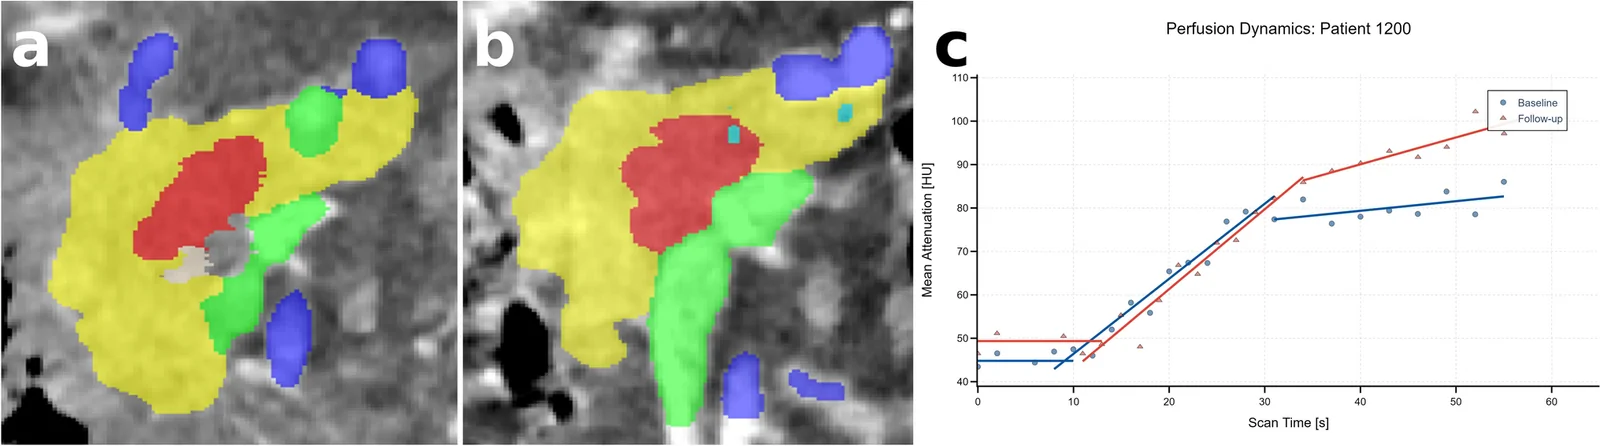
Representative CT perfusion images and analysis. A Baseline CTP image with AI-generated tumor segmentation (red),pancreas parenchyma segmentation (yellow), arteries (green) and vessels (blue). B Follow-up CTP at 3 months with corresponding segmentations. C Time-intensity curves demonstrating increased peak enhancement at follow-up (orange) compared to baseline (blue)
